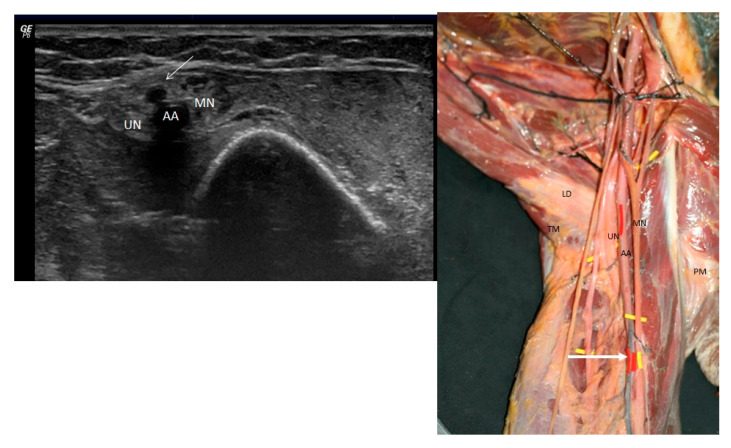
(Left) Ultrasound view of the axillary artery (AA) and the superficial ulnar artery (SUA) marked with a white arrow in relation to the median nerve (MN) and the ulnar nerve (UN). (Right) Dissection of the ulnar nerve (UN) and median nerve (MN) in relation to the axillary artery branches of the brachial plexus and the superficial ulnar artery (white arrow). Pectoral mayor (PM),latissimus dorsi (LD) and teres major muscle (TM).
